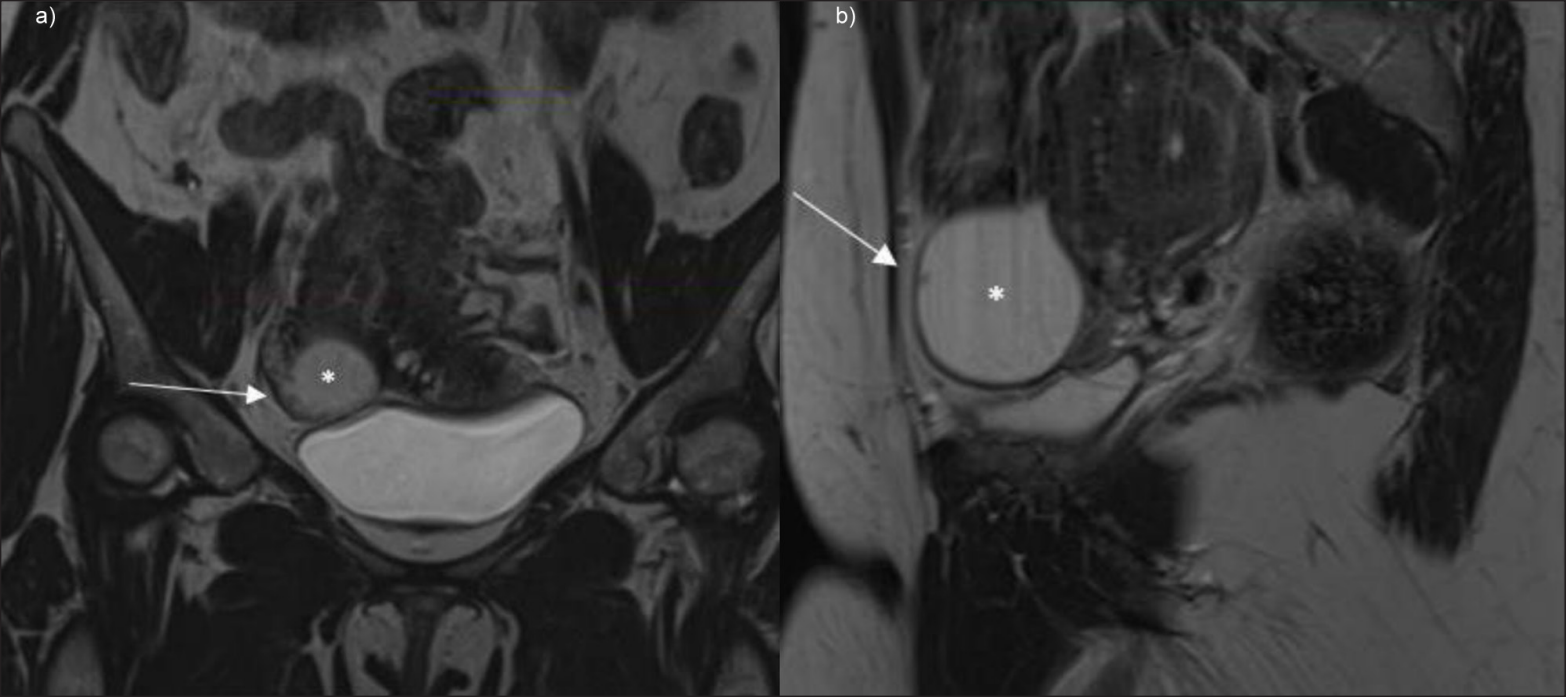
T2-weighted MR (a, coronal, left panel; b, parasagittal, right panel).
